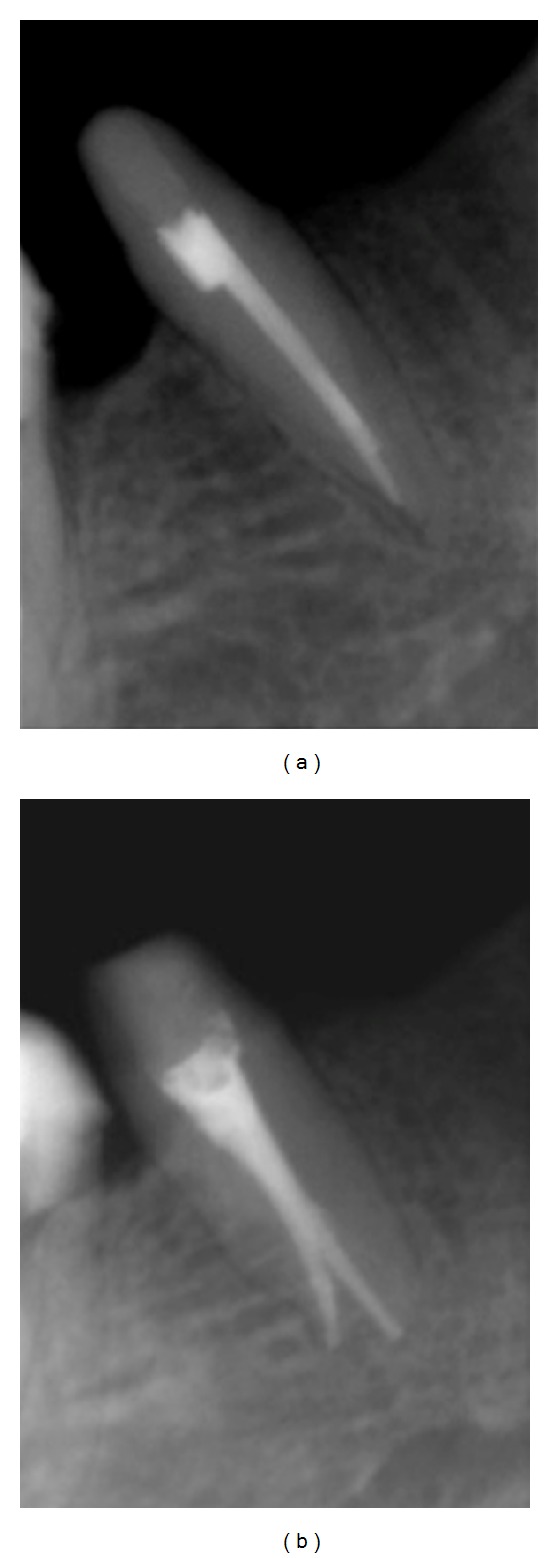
(a) Radiograph in straight angulation. (b) An additional radiograph with a 15–20 degrees horizontal angulation clearly showing a dividing canal.
